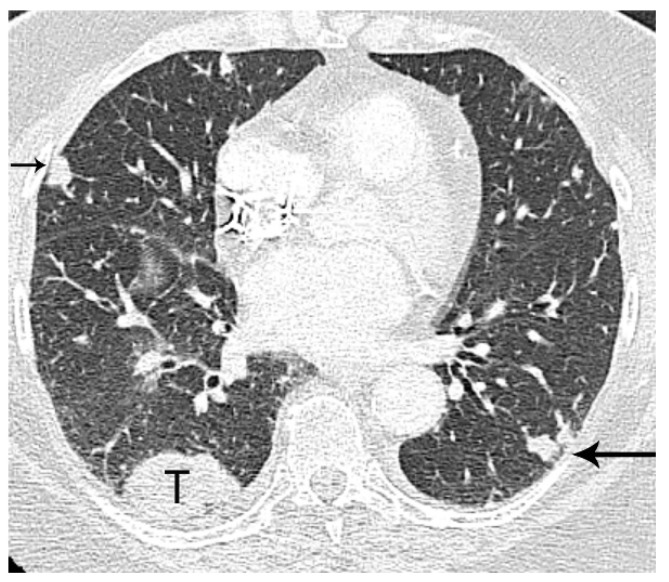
M1a disease, intrathoracic metastasis. Contrast-enhanced CT shows a 4 cm primary tumor (T) in the right lower lobe. Separate nodule in the right middle lobe (short arrow) as the primary tumor is T4 disease. Separate nodule (long arrow) in the left lower lobe (contralateral lung) as the primary tumor is M1a disease.
